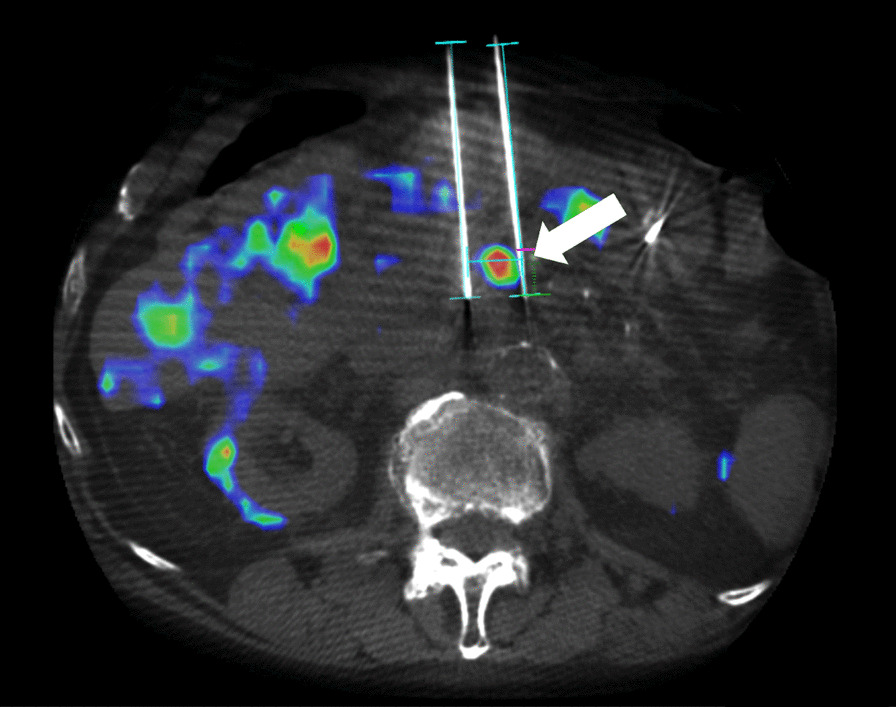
Intraprocedural fused cone-beam CT with preprocedural PET allows for accurate positioning of the IRE electrodes adjacent to the pancreatic body tumor (arrow)
